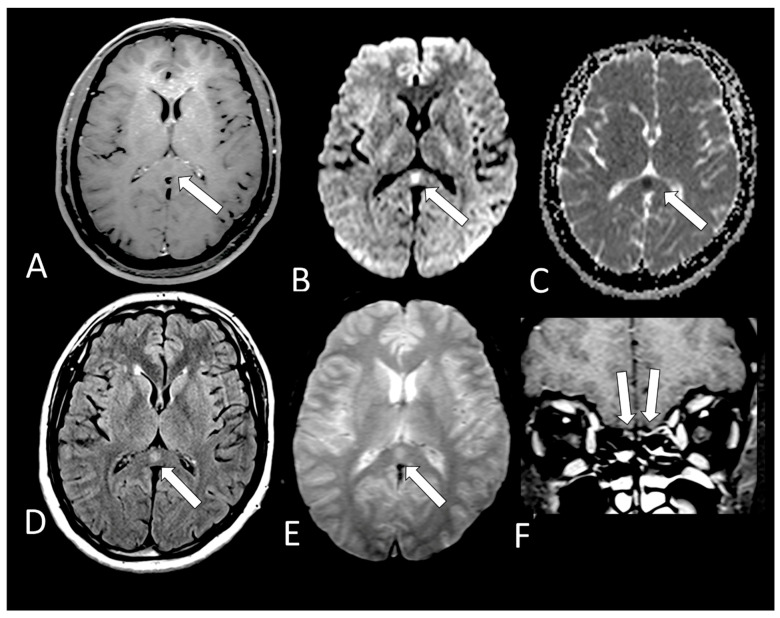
Case 18—On MRI, there is a small round lesion on the splenium of the corpus callosum which could represent a cytotoxic lesion due to a cytokine storm and differential diagnosis is with small acute infarct. It is hypointense on T1 (A, arrow) with restricted diffusion, being hyperintense on DWI (B, arrow) and hypointense on ADC-Map (C, arrow). This lesion is also hyperintense on FLAIR (D, arrow) and T2* (E, arrow) without microbleeding. The olfactory bulbs are hyperintense on coronal post contrast fat suppressedT1WI (F, arrows) in comparison to the gray matter of the frontal lobes, which can represent enhancement, but we cannot exclude microbleeding.
